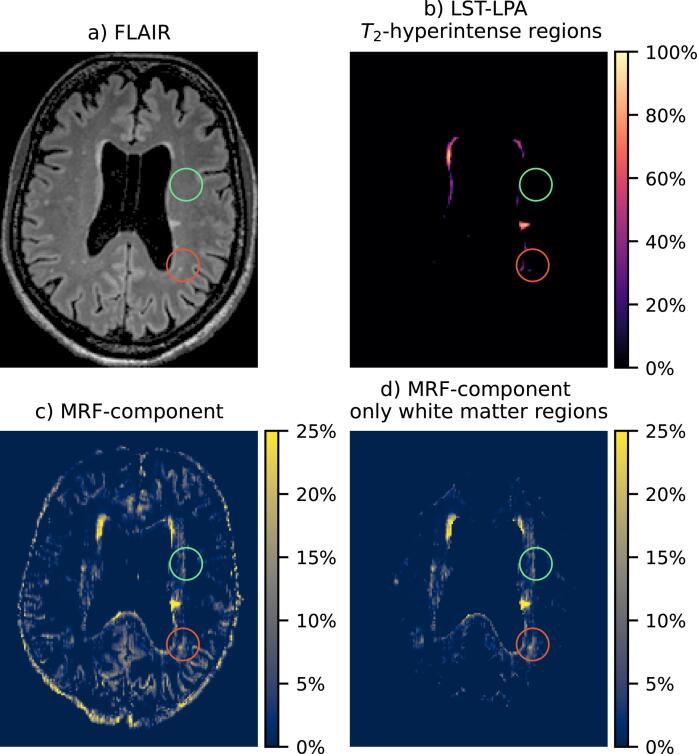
Results from a single slice of a second MS patient. A region with *T*_2_-hyperintense regions on the FLAIR scan is indicated with a red circle; a region reflecting white matter tissue changes visible only on the MRF map is indicated with a green circle. a) FLAIR scan, b) estimated lesion probability map from the FLAIR scan, c) MRF-component with long $T_{2}^{*}$ before masking and d) after masking non-white matter regions. (For interpretation of the references to color in this figure legend, the reader is referred to the web version of this article.)
